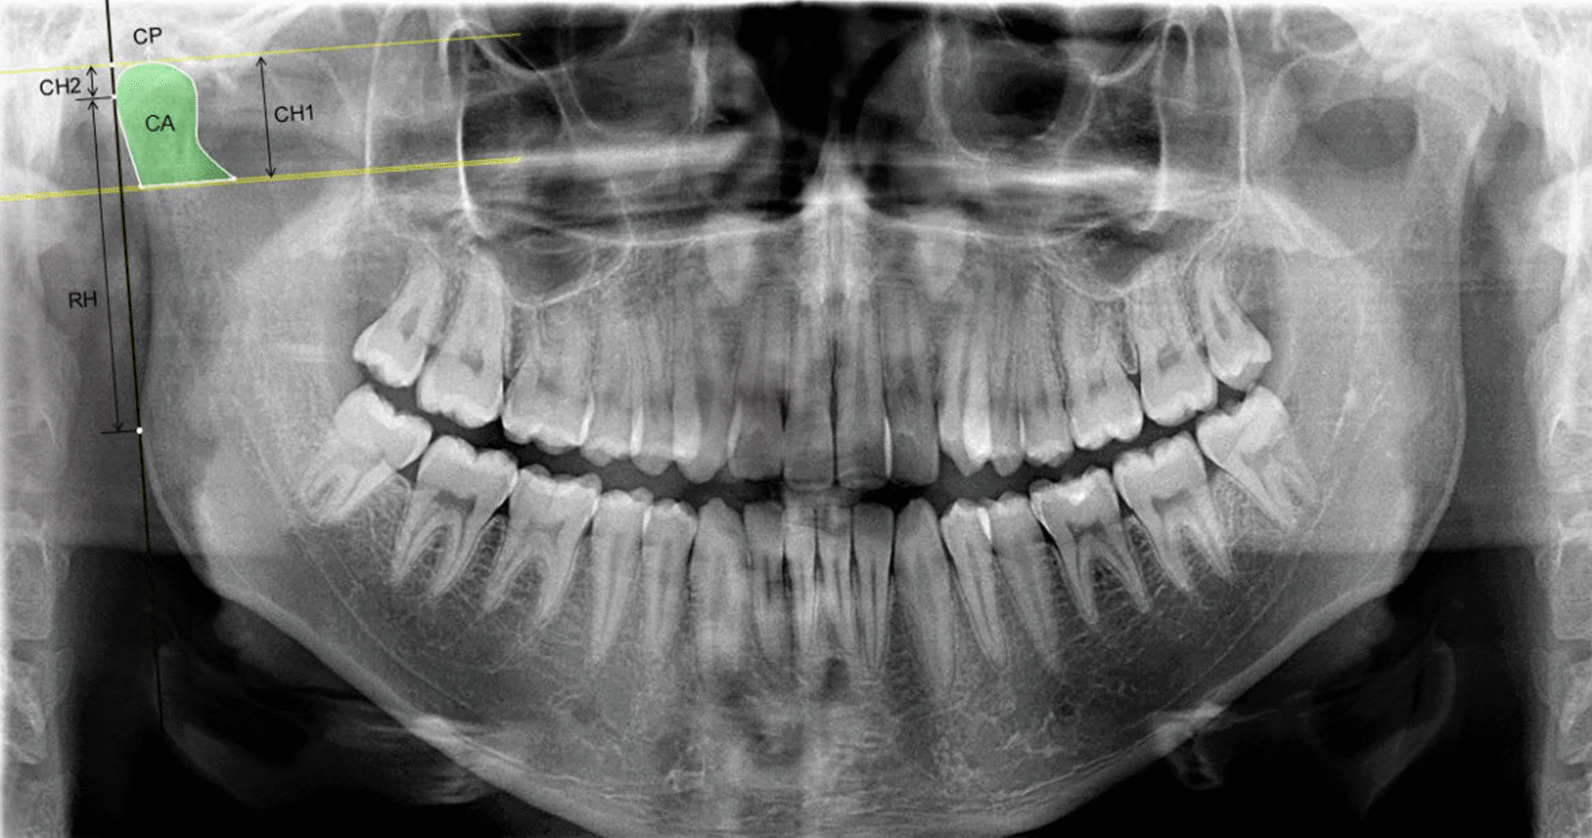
Dental panoramic radiograph showing the selection of the condylar area (CA), the condylar perimeter (CP), the condylar height 1 (CH1), the condylar height 2 (CH2), the ramal height (RH) and the total height (CRH = CH2 + RH)
